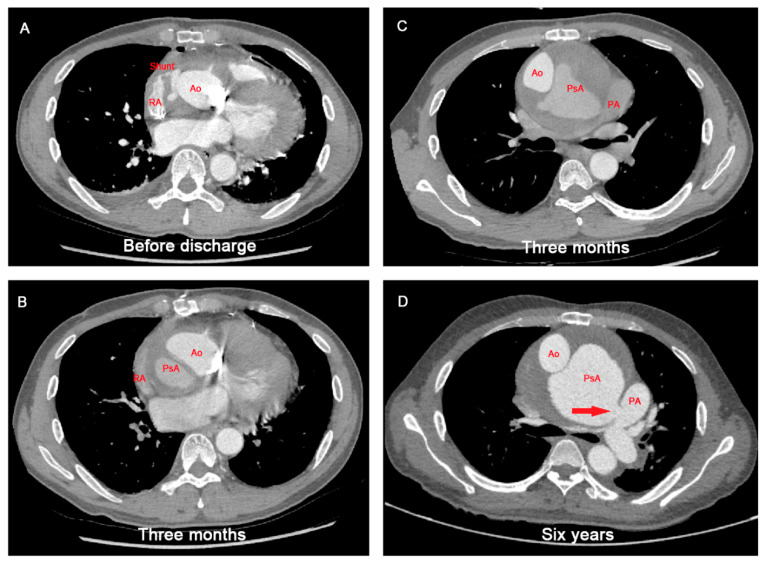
Aortic computed tomographic angiography scan showing the pathogenetic course of pseudoaneurysm and aortopulmonary fistula after Bentall procedure. (A) A patent Cabrol shunt after the initial Bentall procedure. (B,C) Cabrol shunt obliteration and pseudoaneurysm formation 3 months after the initial Bentall procedure. (D) Aortopulmonary fistula formation 6 years after the initial Bentall procedure. Ao: aorta; PA: pulmonary artery; PsA: pseudoaneurysm; RA: right atrium; red arrow indicates the aortopulmonary fistula.
